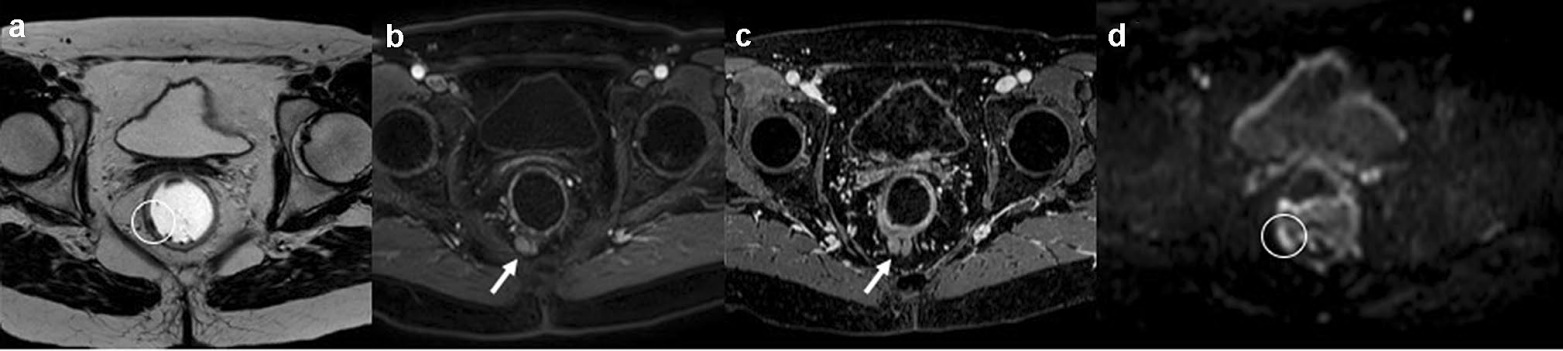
Gastrointestinal neuroendocrine carcinoma of rectum (NEC); MRI axial and coronal T2w imaging (a, e), T2w FAT-SAT, dynamic contrast-enhanced imaging (c) and DwI (d) demonstrate a thickening of right wall of rectum (white arrow and white circle)
